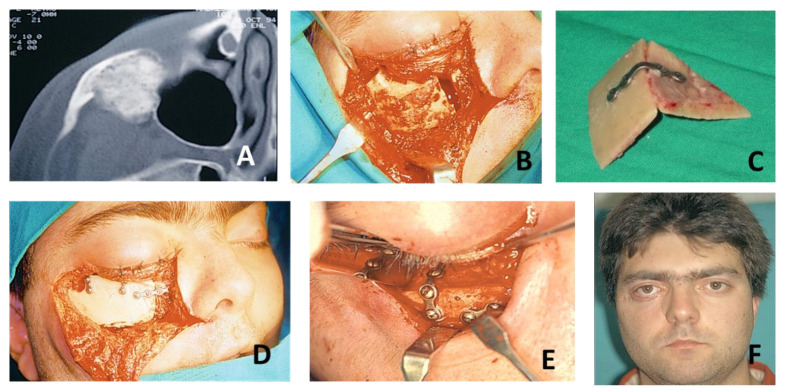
A 55-year-old male was referred from another hospital with a suspected intraosseous vascular malformation in the right zygomatic bone. After CT and MRI studies, the diagnosis was confirmed (A). Surgical treatment was performed using standard technique with a subciliary approach with external canthotomy and lateronasal incision (B) with complete resection of the tumor and primary reconstruction by means of a calotte graft obtained in the same surgical act (C). The calotte graft was adapted to the defect and fixed by means of miniplates and ostesynthesis screws (D,E). During follow-up, the patient reported discomfort in relation to the oteosynthesis material, so it was partially removed. Moreover, he presented lagosphthalmos as sequelae of the approach (F).
